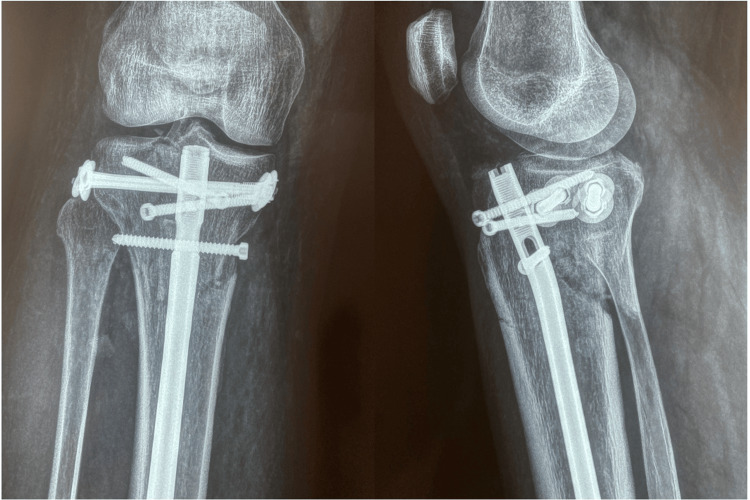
Postoperative X-rays of a patient with a Schatzker type VI tibial plateau fracture treated with the SNB technique SNB: suprapatellar nailing and condylar bolts
